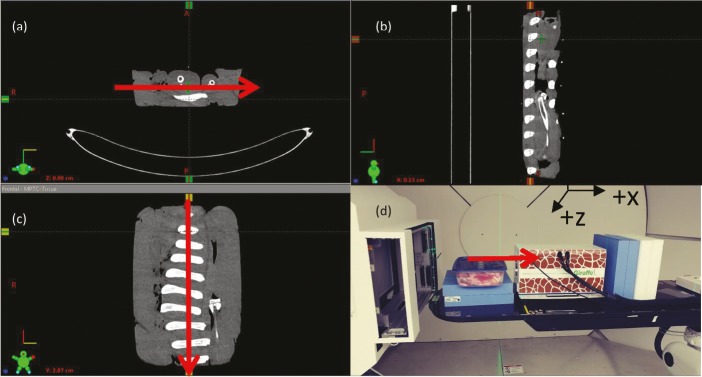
Computed tomography (CT) images of the postoptimized tissue surrogate axis (xy-plane) (a), sagittal (yz-plane) (b), coronal (xz-plane) (c), and measurement setup (d). Red arrows indicate the direction of the proton beam to determine the water-equivalent thickness (WET) value of the postoptimized tissue surrogate. The measured WET value of the tissue surrogate is compared against the treatment planning system WET value to determine the overall uncertainty of the CT calibration curves.
